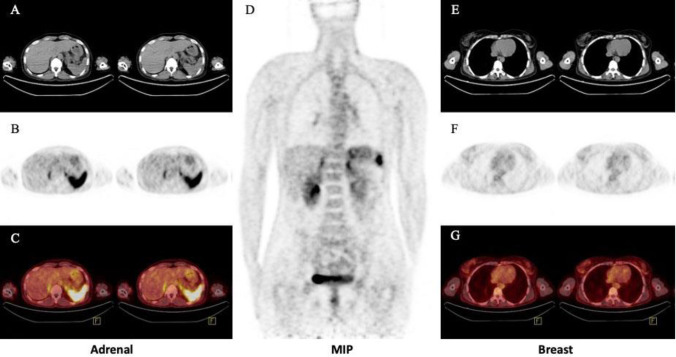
[^68^Ga] Ga-Pentixafor PET/CT. depicting slices of adrenals and breasts normal uptake
